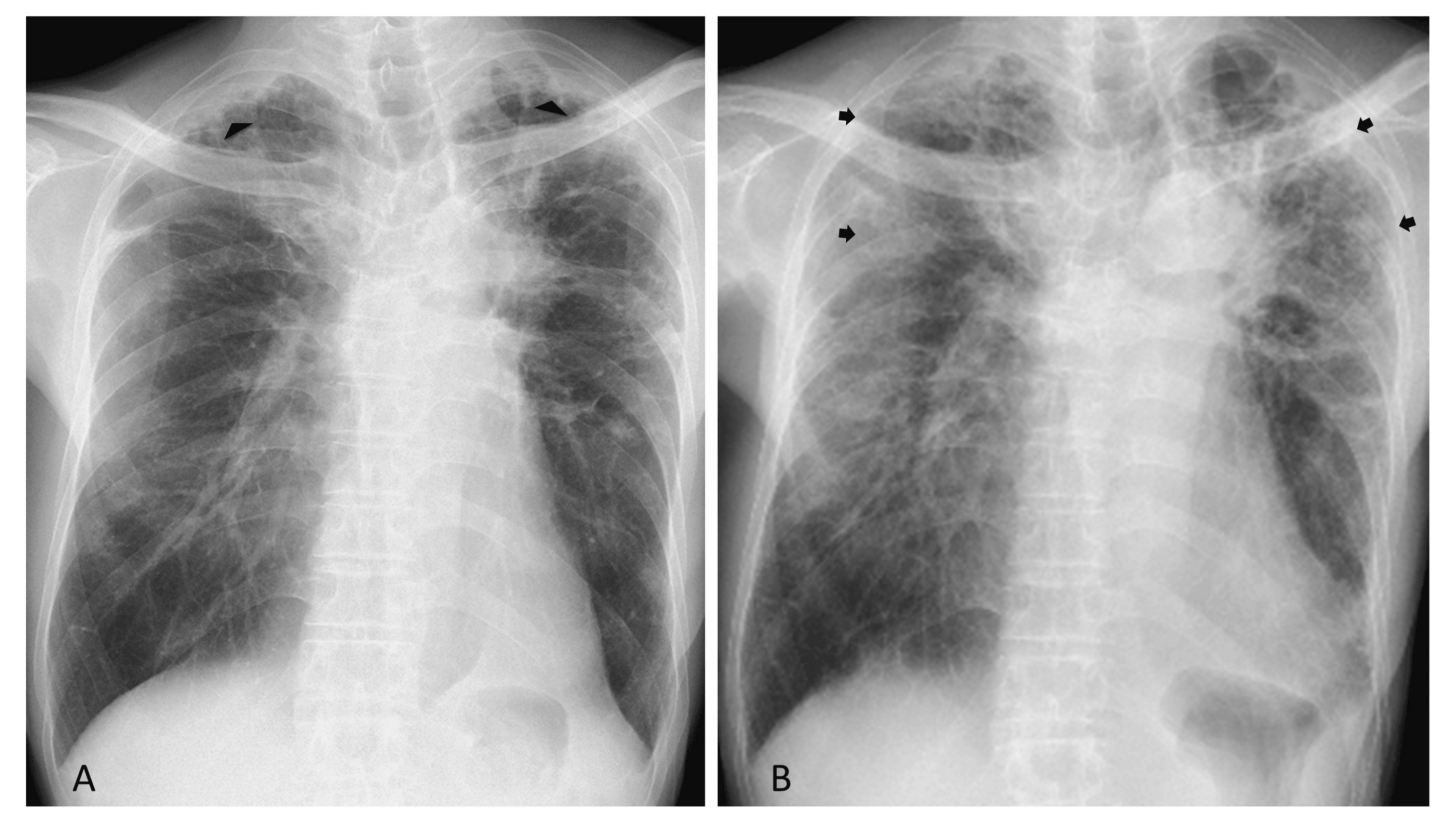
Chest X-ray findings. (A) In May 2018, a chest X-ray revealed irregular apical thickening (arrow heads) and reticular opacities in both upper lung fields. (B) In June 2023, a chest X-ray showed progression of fibrotic changes, with increasing reticular shadows and ground-glass opacities (arrows) in the upper lung fields, accompanied by an upward displacement of the hilar structures and a reduction in the volume of the upper lung lobes.
